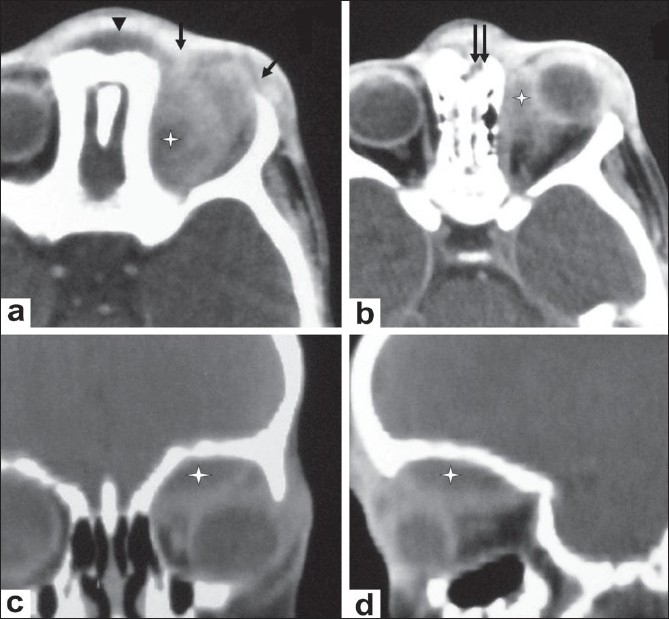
A 1-year-old child presented with mild pain and swelling of the left eye. Axial contrast-enhanced CT scans (a,b) show an extraconal orbital abscess (asterisk in a) with extension into the preseptal tissues (arrows in a) and into the soft tissue of the root of nose (arrowhead in a). There is involvement of adjacent ethmoid sinuses, with irregularity and destruction of their walls. Irregularity and destruction of the orbital plate of the frontal bone and nasion is also seen (double arrow in b). Coronal (c) and sagittal (d) CT scan reconstructions also demonstrate the extraconal orbital abscess (asterisk)
